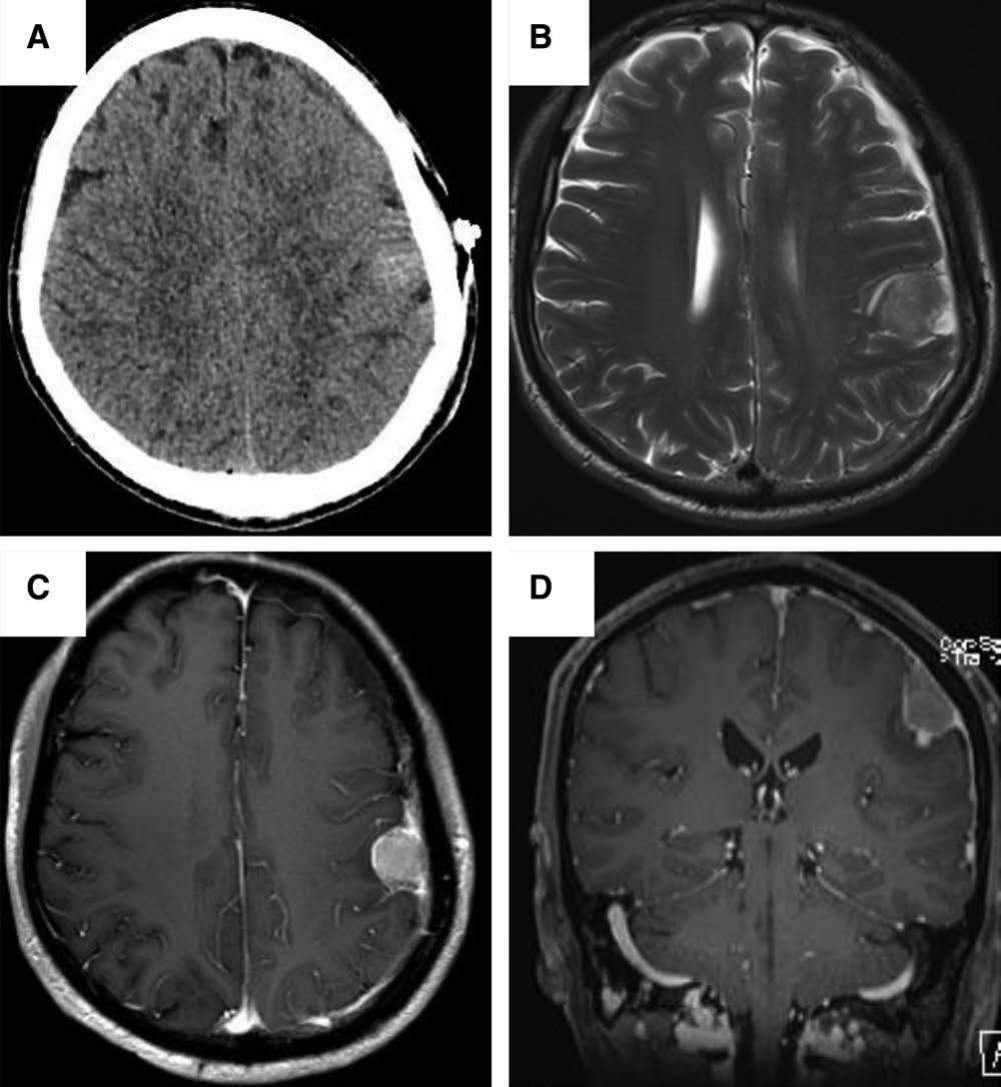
(A) (CT) A mass on the left temporoparietal lobe. (B) (MRI) Dural tail sign and adjacent meningeal thickening. (C and D) (enhanced MRI) Enhancement of the mass, small nodules on the margin. CT = computed tomography, MRI = magnetic resonance imaging.
